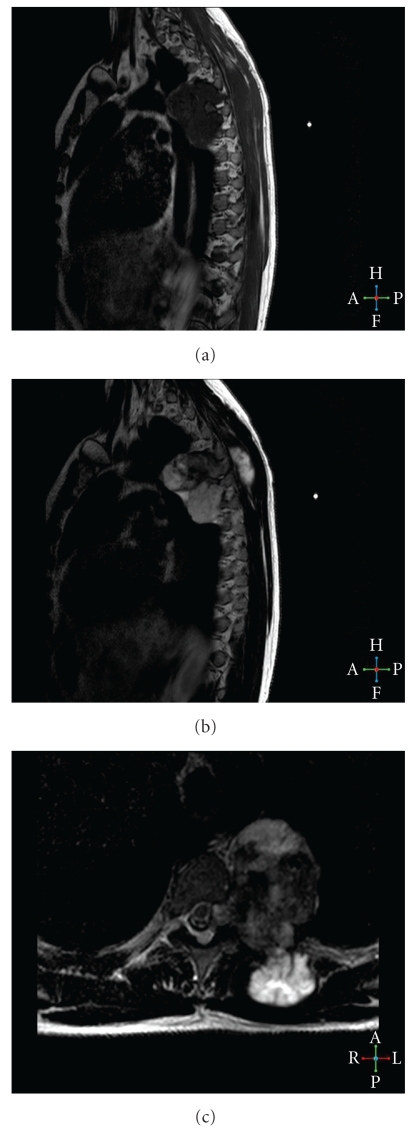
The sagittal T1-w (a) MR image shows a hypointense lobulated lesion. The mass demonstrates heterogeneous but predominantly high signal intensity in the sagittal T2-w. (b) MR image. Note the superficial palpable component of the tumor. (c) The T2 axial view shows how the tumor is positioned next to the ventral structures and its relationship to the spinal cord.
